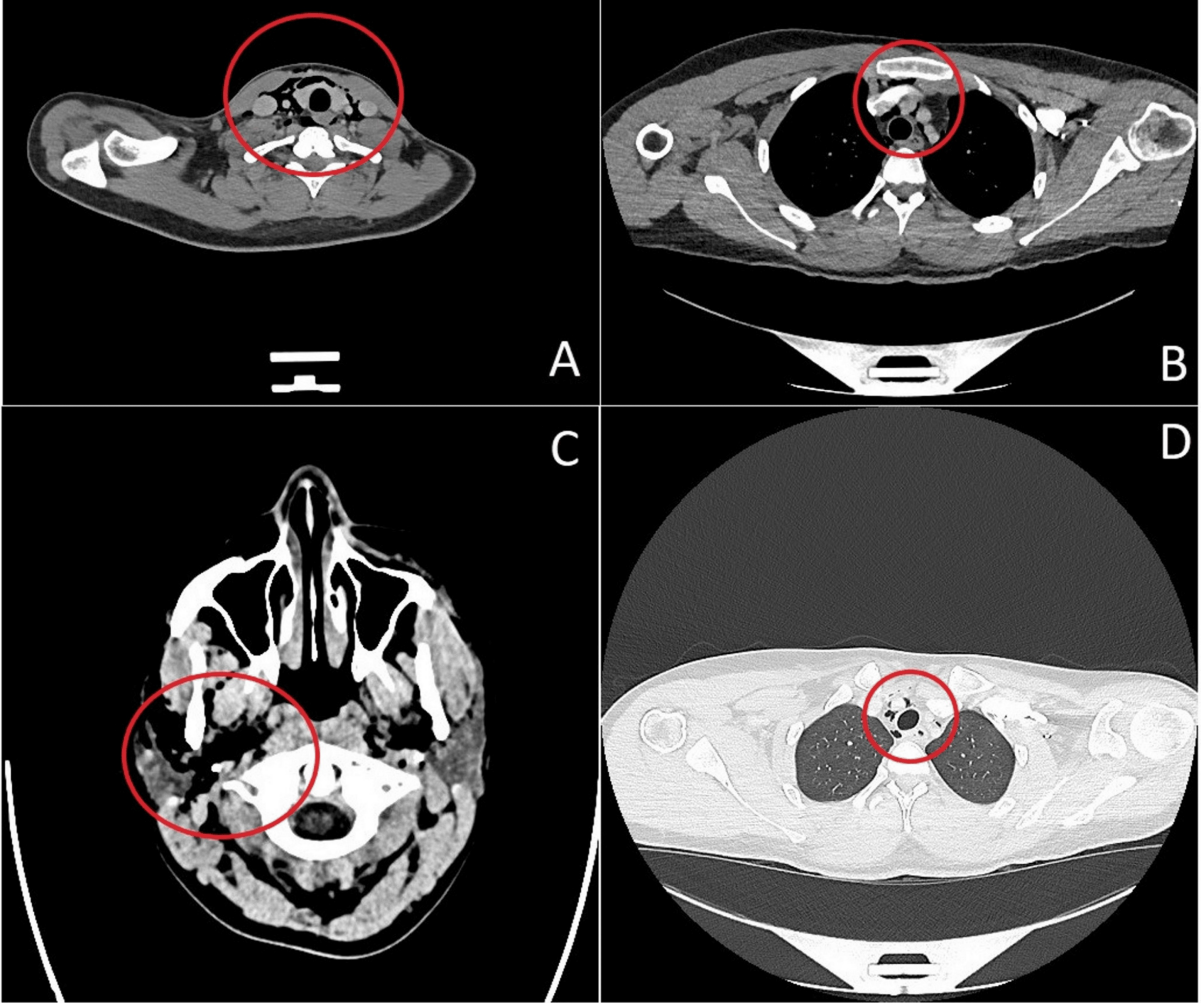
CT scan at second presentation at the emergency care (A) Upper thoracic imaging in soft tissue window, (B) thoracic imaging in soft tissue window, (C) CT scan of the skull in soft tissue window, and (D) thoracic imaging in lung window CT: computed tomography
